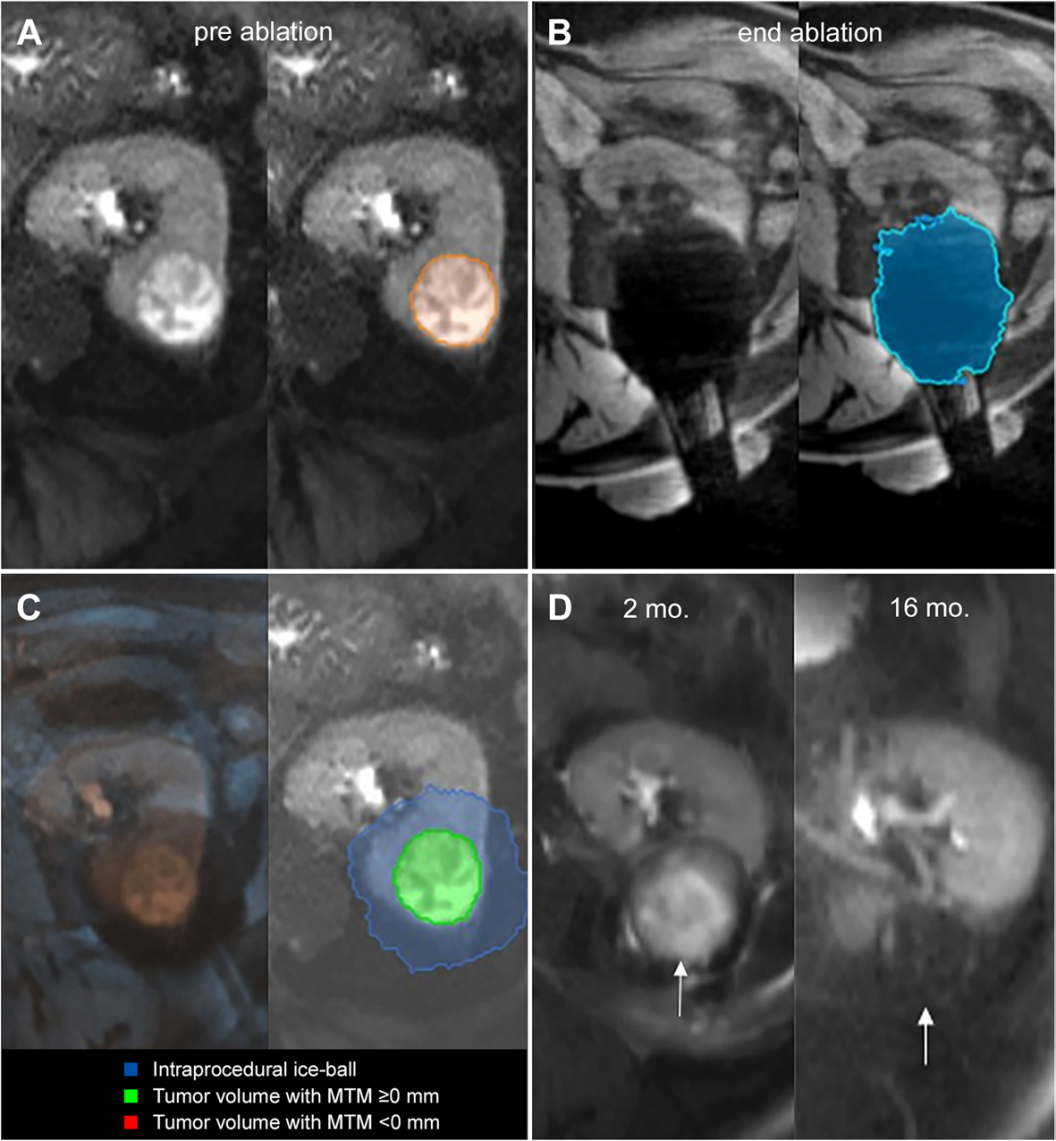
A Segmentation (orange outline) of a 3.5 cm diameter tumor located interpolar in the left kidney on pre-ablation axial T2-weighted MR imaging. B Segmentation (blue outline) of corresponding end ablation ice-ball volume on post-ablation axial T1-weighted MR imaging. C Overlay of pre-ablation and post-ablation images after co-registration (left) and resulting 3D margin calculation (right). Volumetric assessment shows complete tumor coverage (green) by the end-ablation ice-ablation ice-ball (blue) with MTM of 4 mm. D Ablation zone (white arrows) without tumor recurrence on follow-up axial T2-weighted MR imaging 2 months (left) and 16 months (right) after cryoablation
